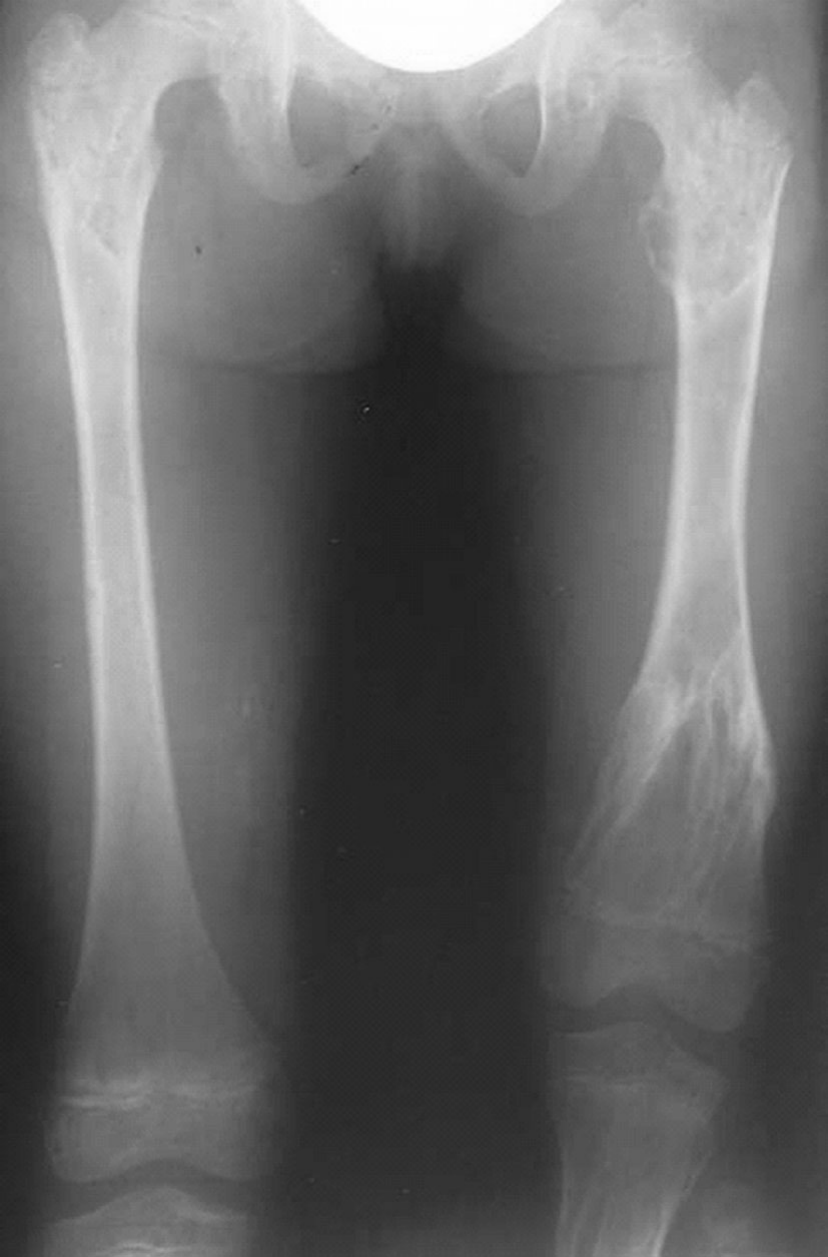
Radiograph showing *left* femur enchondroma in a child with Ollier’s disease
